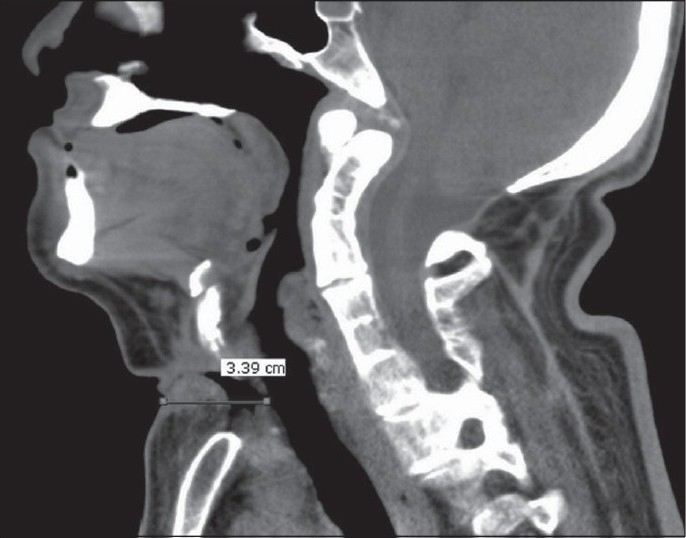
MRI neck lateral view showing thick and short neck with restricted neck extension due to fused cervical vertebrae and the depth of the trachea from skin level to be 3.4 cm
